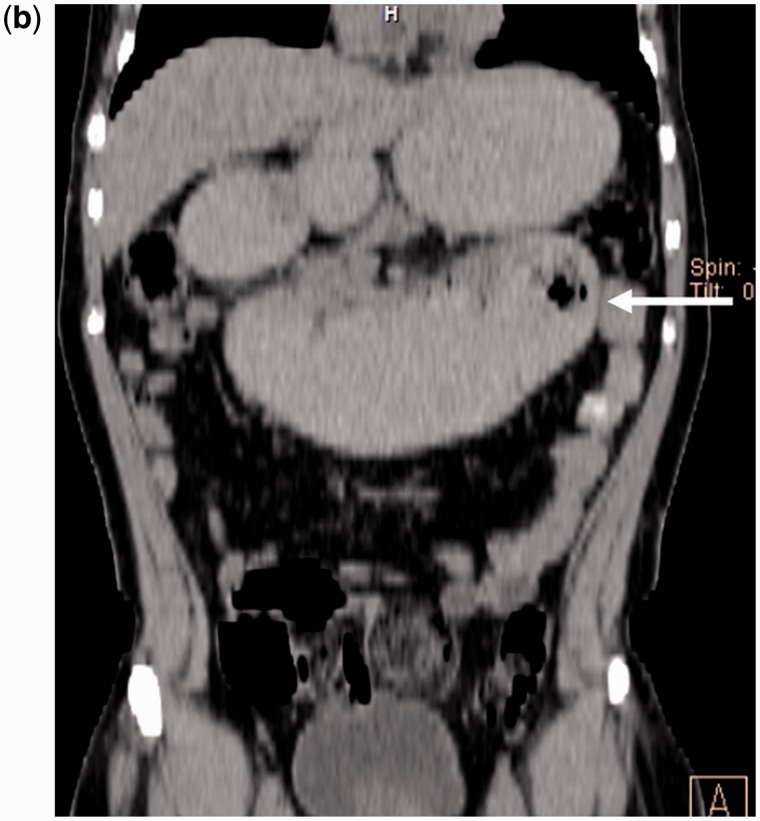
Abdomen CT scan (coronal plane): the area indicated by the arrow showed grossly dilated D1, D2 and D3 segments of the duodenum, with constriction seen at the duodeno-jejunal junction. The small bowel loops are partially collapsed after the lesion.
